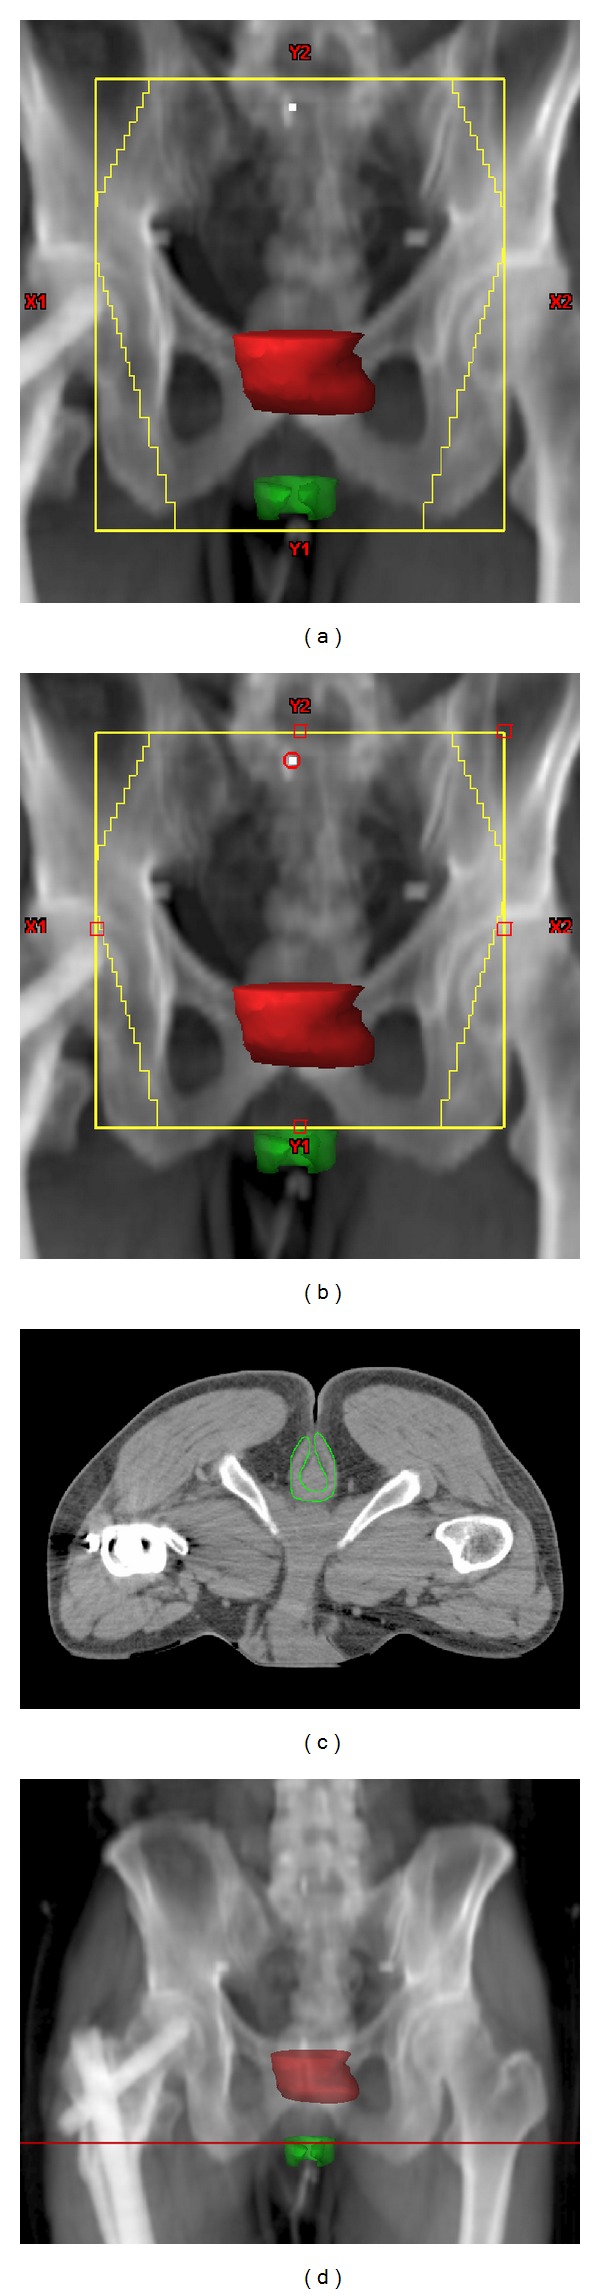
A patient with a rectal cancer located 2.7 cm above the EAS. (a) The original RT field covered 4 cm inferior to the gross tumor. (b) The EAS can be spared from radiation treatment easily by reducing the inferior border superiorly by 2 cm. (c) Axial CT image through the EAS shows that no draining lymph nodes need to be covered. (d) Coronal scout view shows the level of axial image (red line). Solid red: gross tumor; green: EAS.
